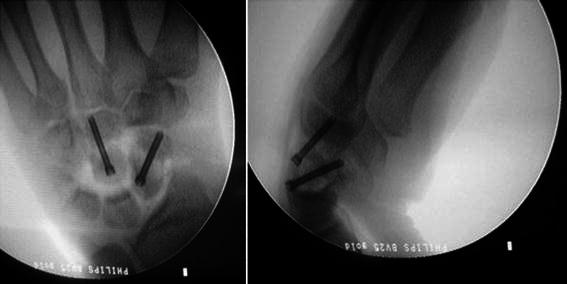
Intraoperative radiograph showing reduction and screw fixation of the scaphoid and capitate. The capitate appears to be aligned with the lunate on the lateral view
